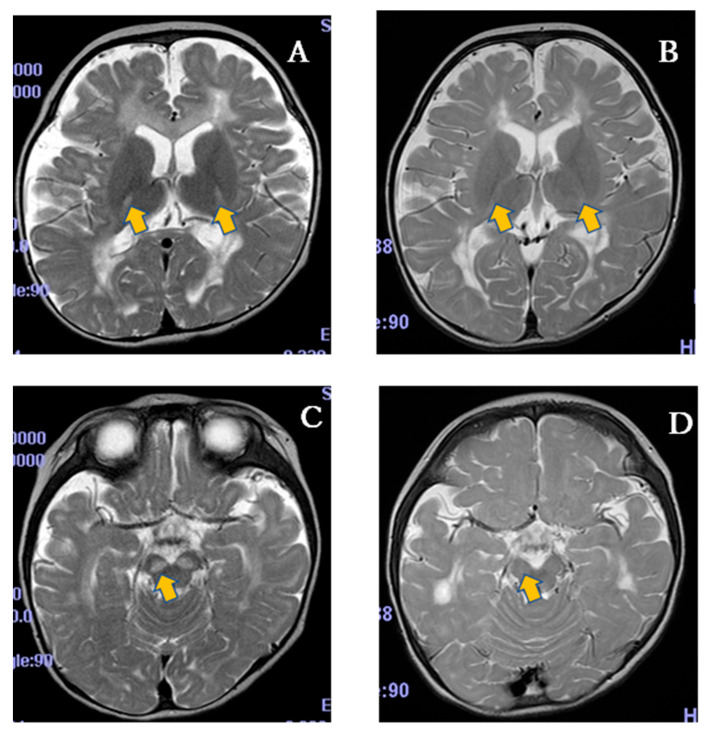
Magnetic resonance imaging of the brain. PLA-04-01 at 19 months old before entering the trial (A,C) and at 36 months old after treatment with 5-ALA and SFC for 17 months (B,D). T2-weighted MRI showed high signal intensity in the bilateral basal ganglia (lateral thalamus) and brainstem (substantia nigra) before the trial (A,C), which decreased after the trial (B,D). The arrows indicate those legions before and after the trial.
